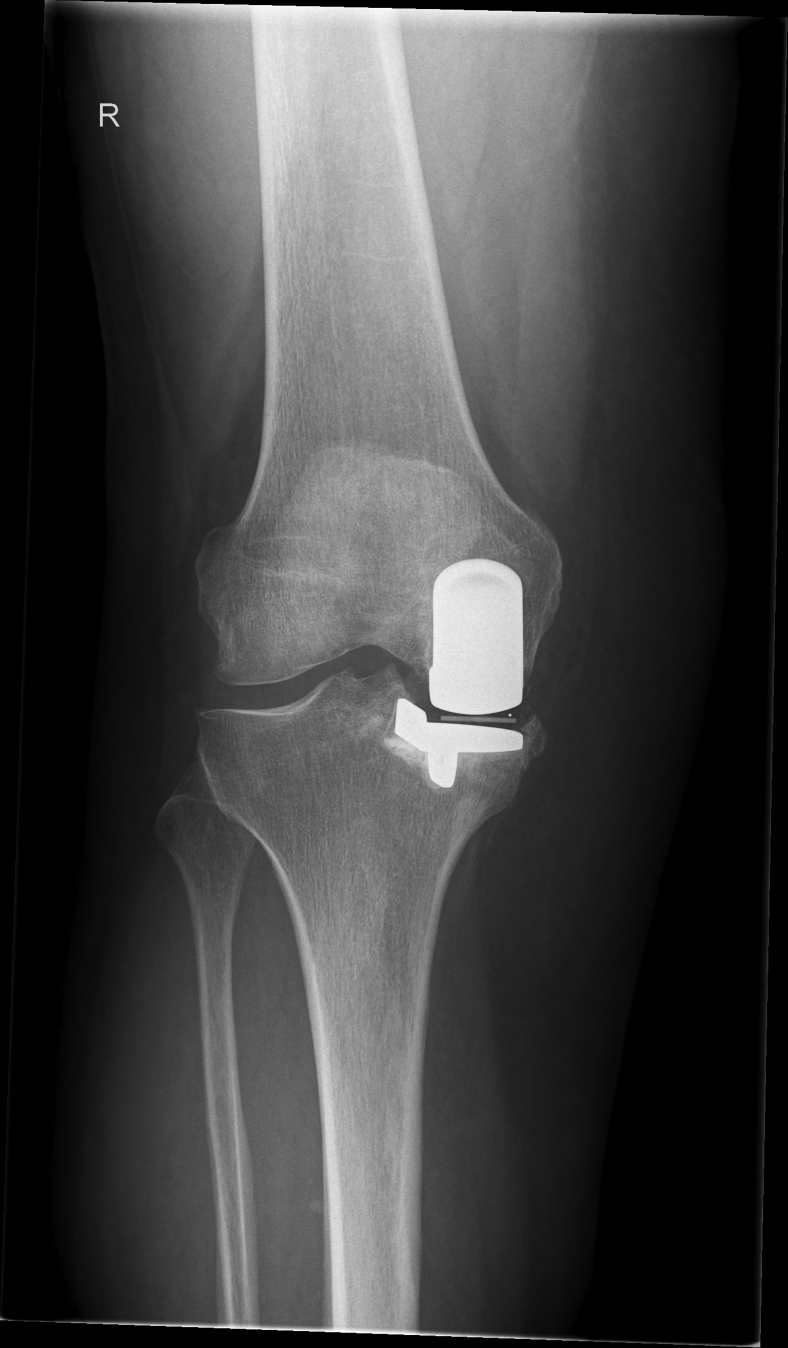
Preoperative anteroposterior radiograph showing the unicompartmental knee arthroplasty.
